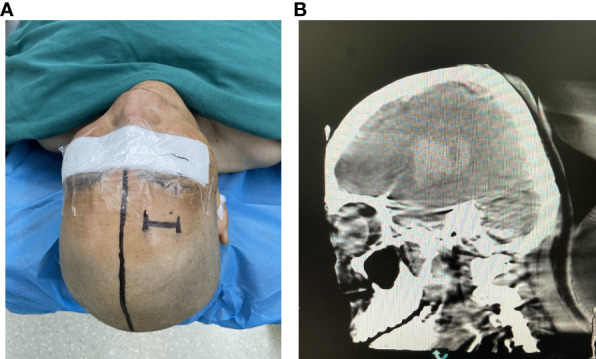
(A) The percutaneous puncture point was marked. (B) Confirming the puncture point in the CT image.
